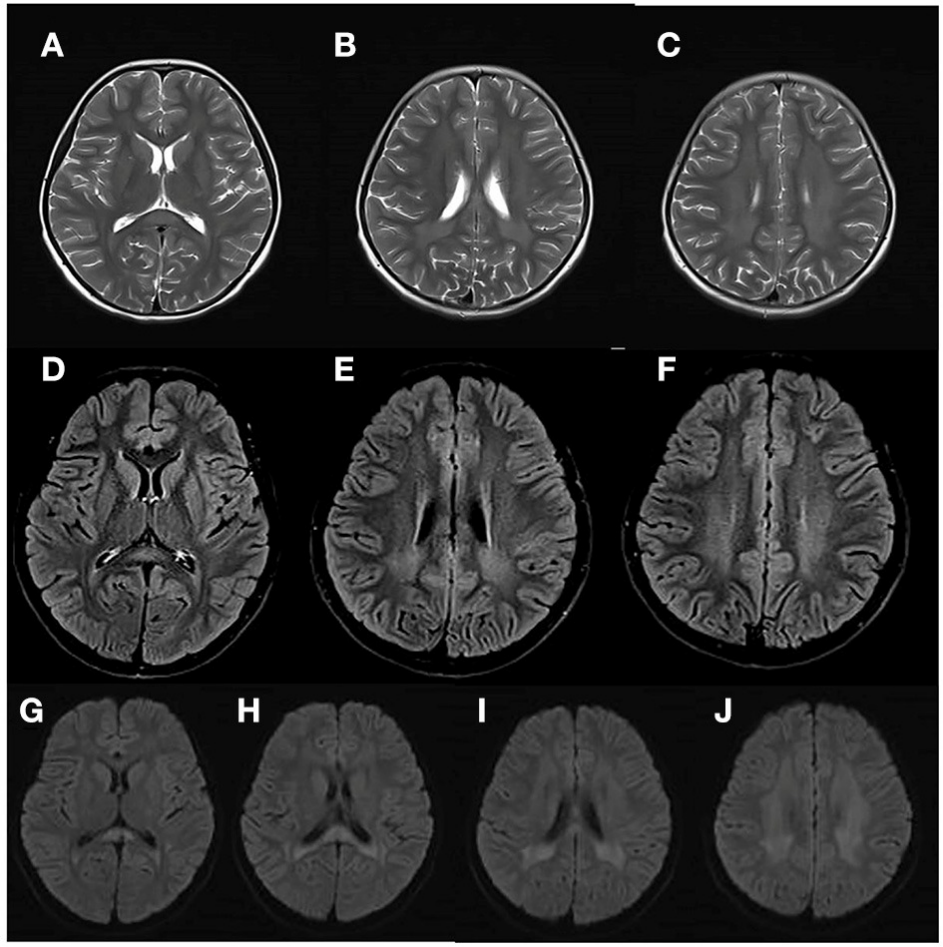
Brain MRI of the patient at her first episode of disease, at 8 years and 9 months. The white matter (WM) showed hyperintense in the T2-weighted axial (A–C) and FLAIR axial (D–F) views, and restricted diffusion in the diffusion-weighted imaging (G–J).
